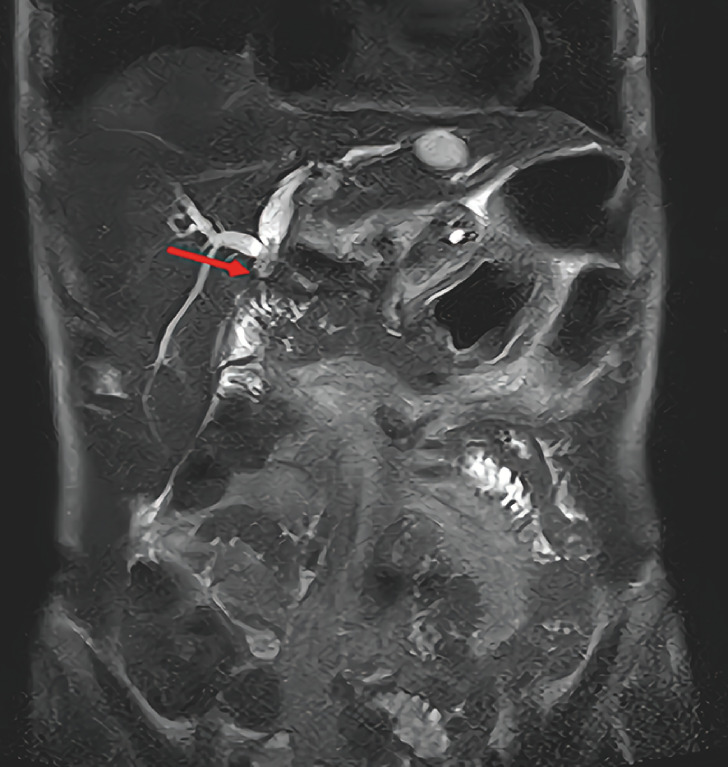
Magnetic resonance imaging showing the dilated and blocked left hepatic duct (red arrow) of a 57-year-old man with recurrent abdominal pain.
